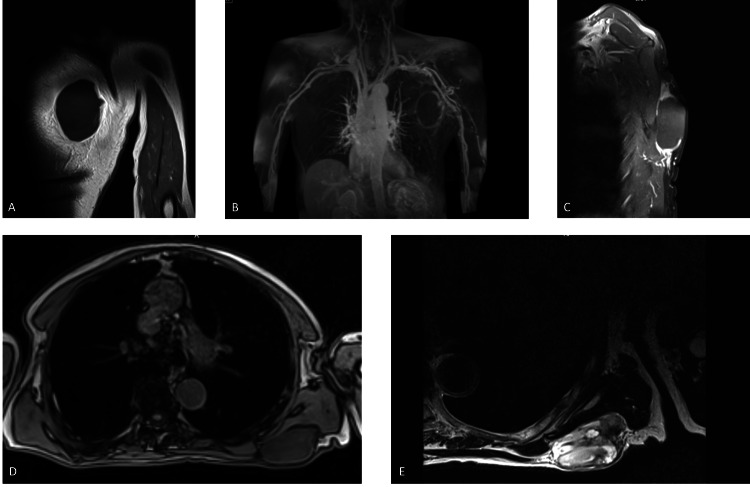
Intravenous contrast-enhanced magnetic resonance imaging. A: T1-weighted turbo spin-echo coronal section of the intravenous contrast-enhanced magnetic resonance imaging performed for the patient’s mass. B: T1-weighted fat suppression coronal section of the intravenous contrast-enhanced magnetic resonance imaging performed for the patient’s mass. C: T1-weighted turbo spin-echo fat suppression sagittal section of the intravenous contrast-enhanced magnetic resonance imaging performed for the patient’s mass. D: T1-weighted transverse section of the intravenous contrast-enhanced magnetic resonance imaging performed for the patient’s mass. E: T2-weighted transverse section of the intravenous contrast-enhanced magnetic resonance imaging performed for the patient’s mass.
